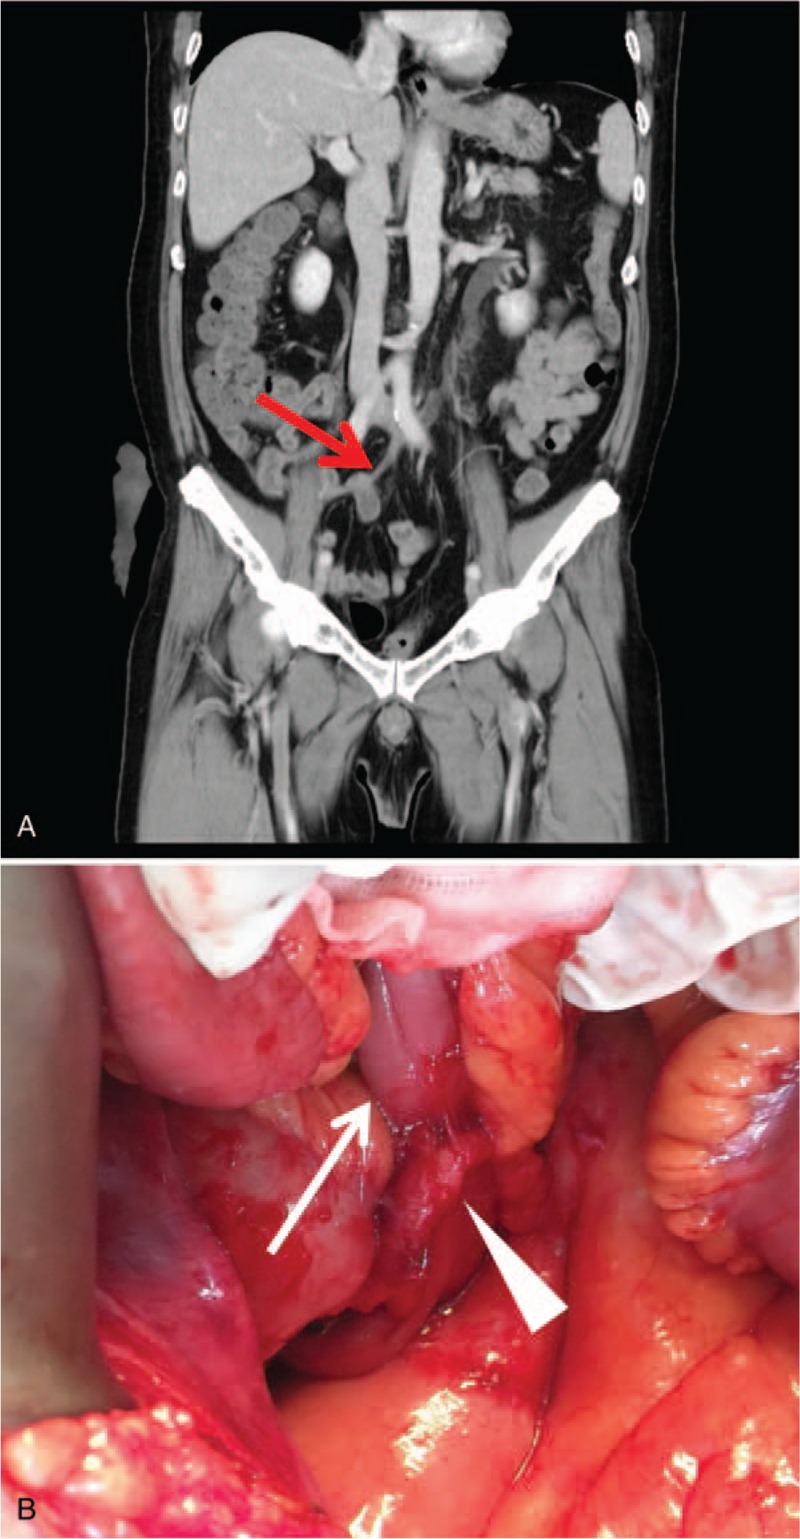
(A) Abdominal CT revealed 1 ureter stricture segment that caused hydronephrosis and hydroureter (arrow: ureter stricture). (B) During exploratory laparotomy, a segment of herniated bowel loop between bilateral ureters was found. The left ureter was complete surrounded by the small bowel and severe adhesion was noted. After complete ureterolysis, a 10Fr nasogastric tube could pass through the ureter smoothly. (Arrow: hernia loop, arrowhead: left ureter).
